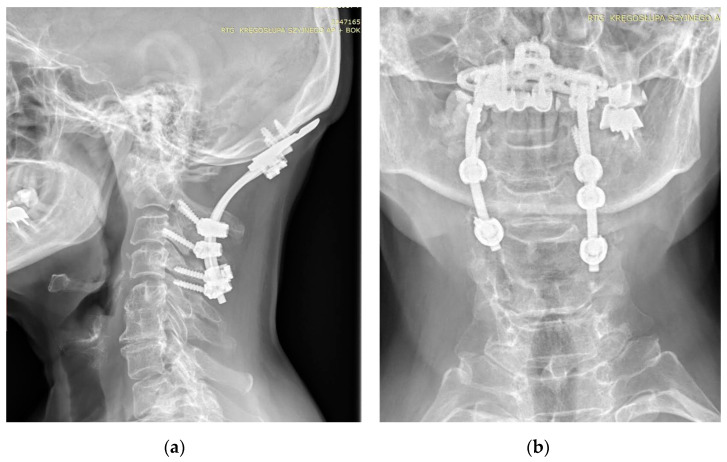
AP (a) and lateral (b) X-rays of the neck of a 67-year-old patient with rheumatoid arthritis and atlantoaxial subluxation, CrS, and ankylosis C5-C6. X-ray showing occipitocervical fusion with C1 laminectomy. Due to anatomical deformities in the course of RA, asymmetric screw placement was possible.
